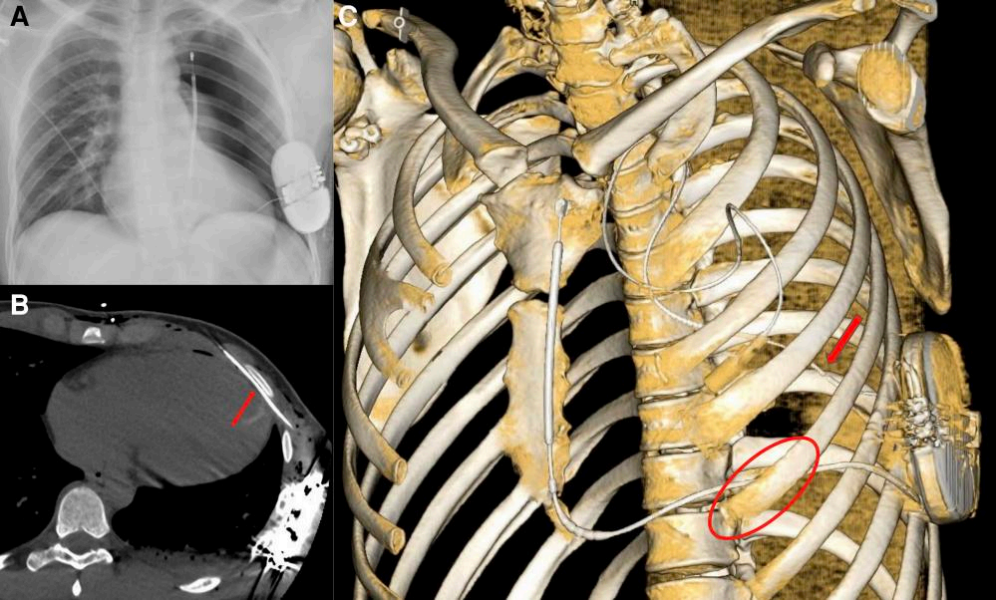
(*A*) Chest X-ray showing the complete left pneumothorax. (*B*) A computed tomography (CT) scan showing the intrapleural pathway of the lead under the 5th rib. (*C*) 3D reconstruction of the CT scan showing the subcostal path of the lead (red circle) and the thoracic drain (red arrow).
